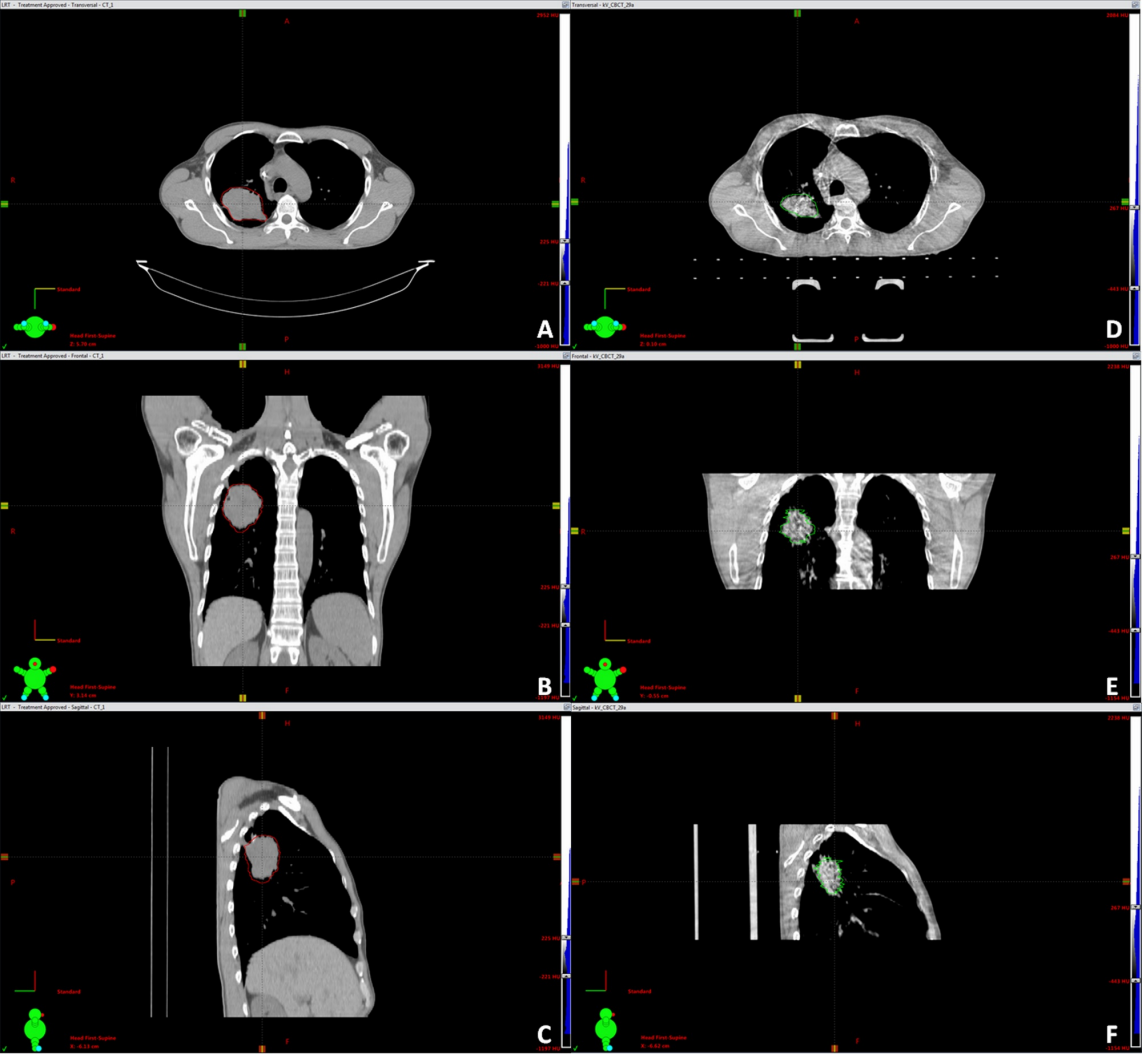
Adaptive therapy because of significant tumor response during treatment An 87-year-old male patient with non-small cell lung cancer (NSCLC) initially treated with GammaKnife (GK; Elekta Instrument AB, Stockholm) radiosurgery for brain metastases three months prior to lattice radiotherapy (LRT) and RT to the primary left upper lobe (LUL) tumor. The tumor volume decreased by 45%. Figures 3A, 3B, 3C: planning CT images in the axial (A), coronal (B), and sagittal views (C) before LRT with GTV = 255.55 cc. Figures 3D, 3E, 3F: New planning computed tomography (CT) images obtained in the axial (D), coronal (E), and sagittal views (F) for adaptive therapy planning necessitated after a significant decrease in volume to 139.6 cc after LRT and 18 conventional fractions. The patient is alive with the disease in a 24-month follow-up.
